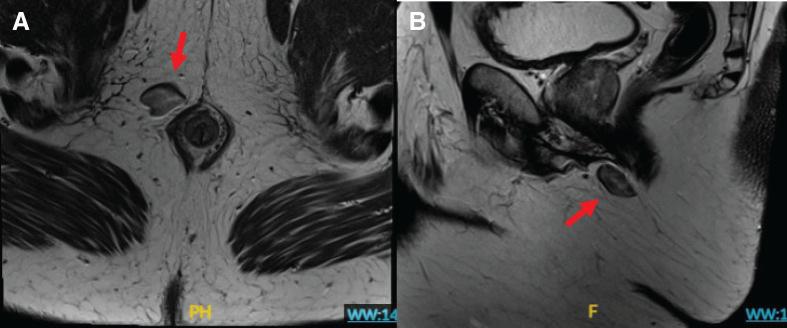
(A) Axial and (B) Sagittal slices of MRI rectum highlighting ~2.1 × 2.7 × 1.8 cm non-specific solid-cystic lesion in the right ischiorectal fossa just superior to the anal verge.
